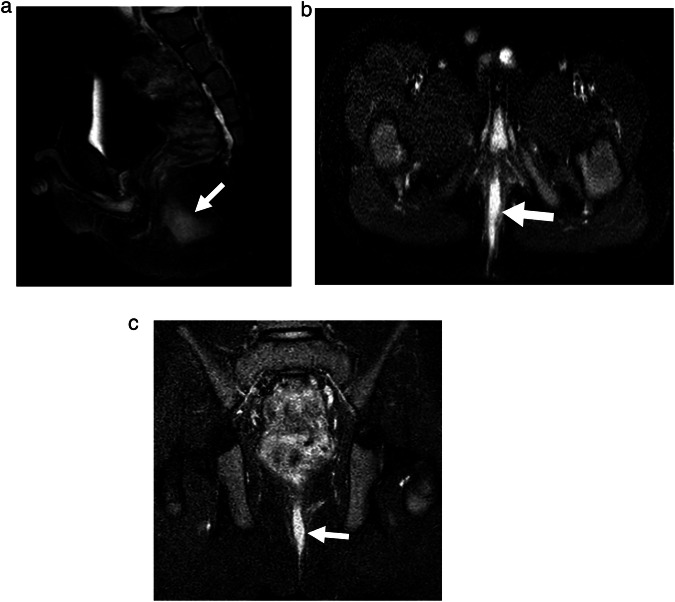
6-year-old boy with perianal Crohn’s disease. MRI pulse sequences include (a) sagittal T2-weighted (T2W) fast spin-echo (FSE) fat-saturated (FS), (b) axial T2W FSE FS, and (c) coronal short tau inversion recovery (STIR). White arrows demonstrate posterior midline linear hyperintense signal abnormality, consistent with a perianal fistula. When using the Parks classification, system, five radiologists described this fistula tract as trans-sphincteric, five described it as intersphincteric, and three described it as suprasphincteric
